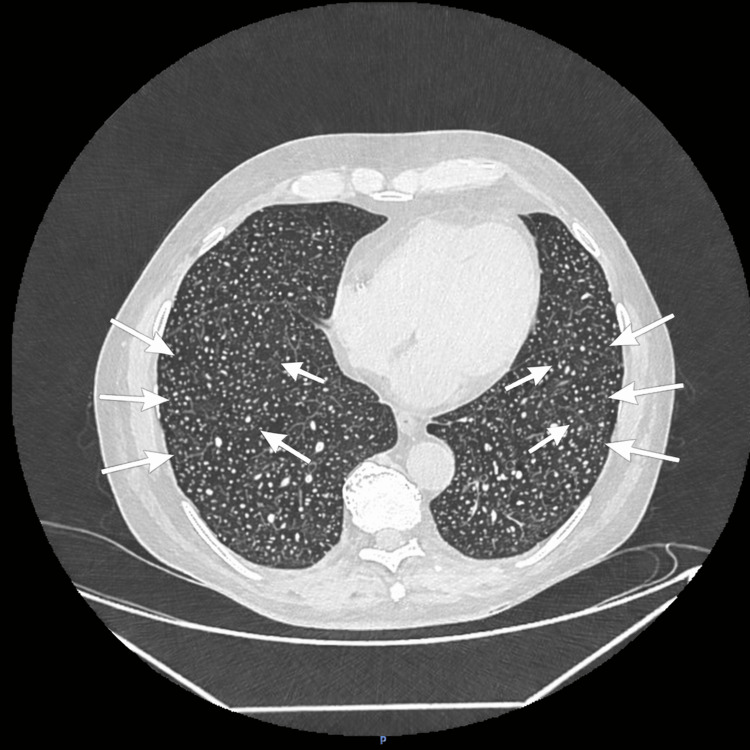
Miliary tuberculosis on the chest computed tomography scan
